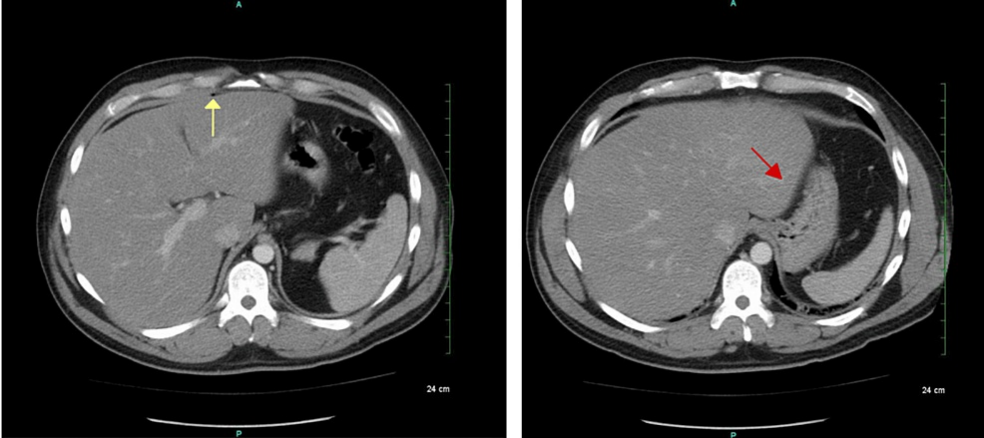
Computed tomography (CT) with intravenous (IV) contrast demonstrating multiple pockets of free air in the midline anterior abdominal wall and peritoneal cavity (yellow arrow). Air pocket tracking toward the liver margin with mesenteric induration and thickening (red arrow)
